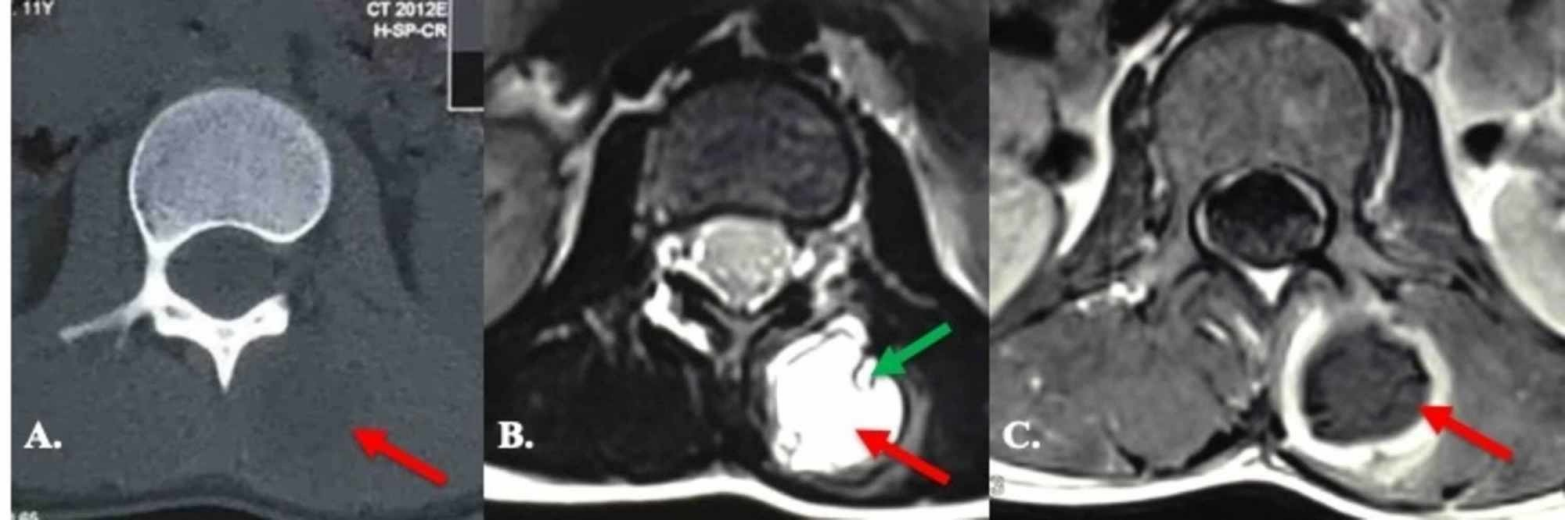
Imaging A) CT scan; B) T2-weighted MRI; C) Contrast-enhanced T1 MRI of the lesion. The red arrow shows the cyst itself while the green arrow shows a daughter cyst.
